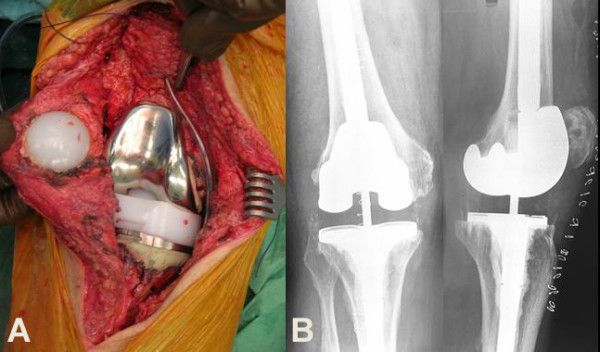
(A) Intraoperative photograph after tricompartmental revision knee arthroplasty. (B) Postoperative anteroposterior (left) and lateral (right) radiographs of the right knee.
